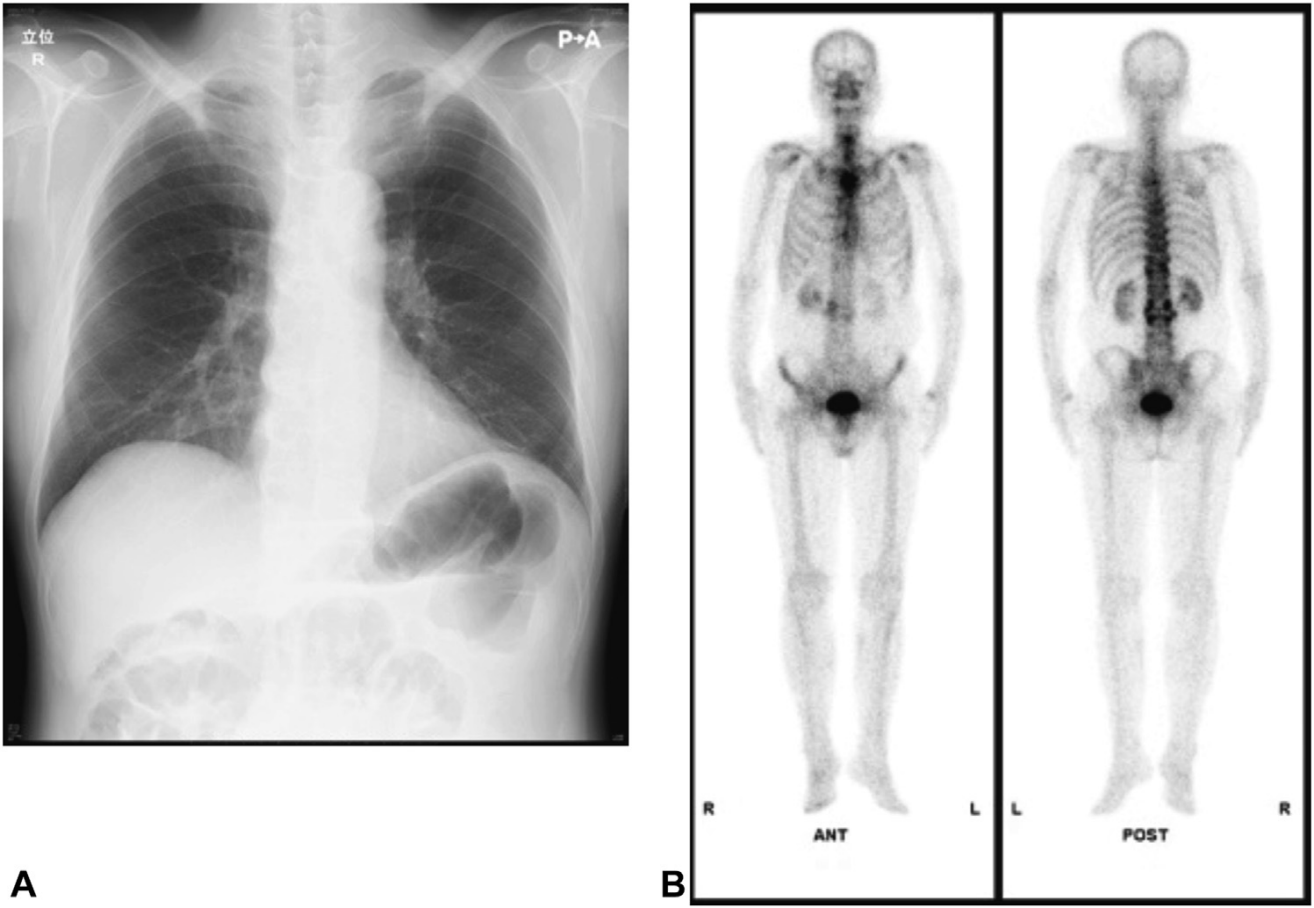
Chest X-ray showed bony hyperplasia of the sternoclavicular joint (A). Bone scintigraphy showed osteosclerosis changes were seen in the bilateral sternoclavicular joints, sternal pattern, and the first and second sternal rib joints and sclerotic changes in the anterior surfaces of the cervical to lumbar vertebral bodies (B).
